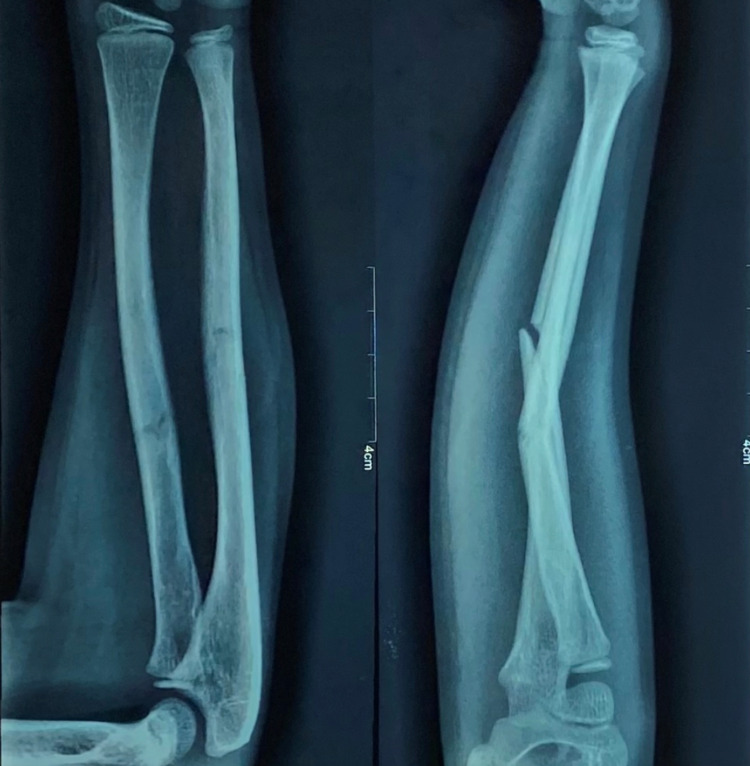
Pre-operative radiograph of a case of radius and ulna shaft fracture
